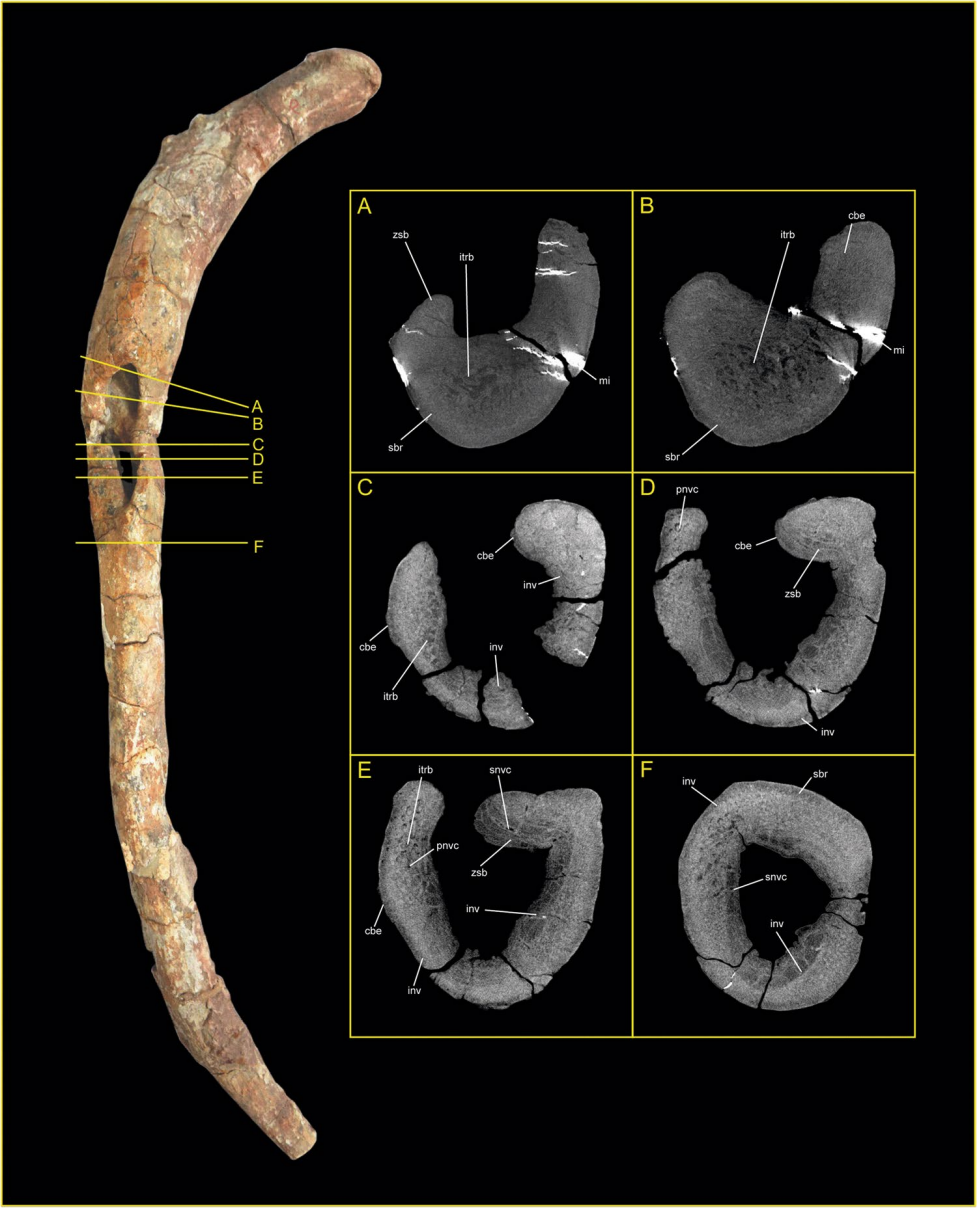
Transverse orthoslices produced from micro-computed tomography of the YX V0003 rib. Each slice represents an aligned transverse x-ray slice taken perpendicular to the cortical surface of the bone at the locations marked A to F in Fig. 4. Abbreviations: cbe = cortical bone expansion; inv = involcrum; itrb = internal trabeculae; pnvc = primary neurovascular canal; sbr = sequestered bone region; snvc = secondary neurovascular canal; zsb = zone of sclerotized bone. Photograph by Lida Xing. Orthoslices produced by P.S.R.-Q.
